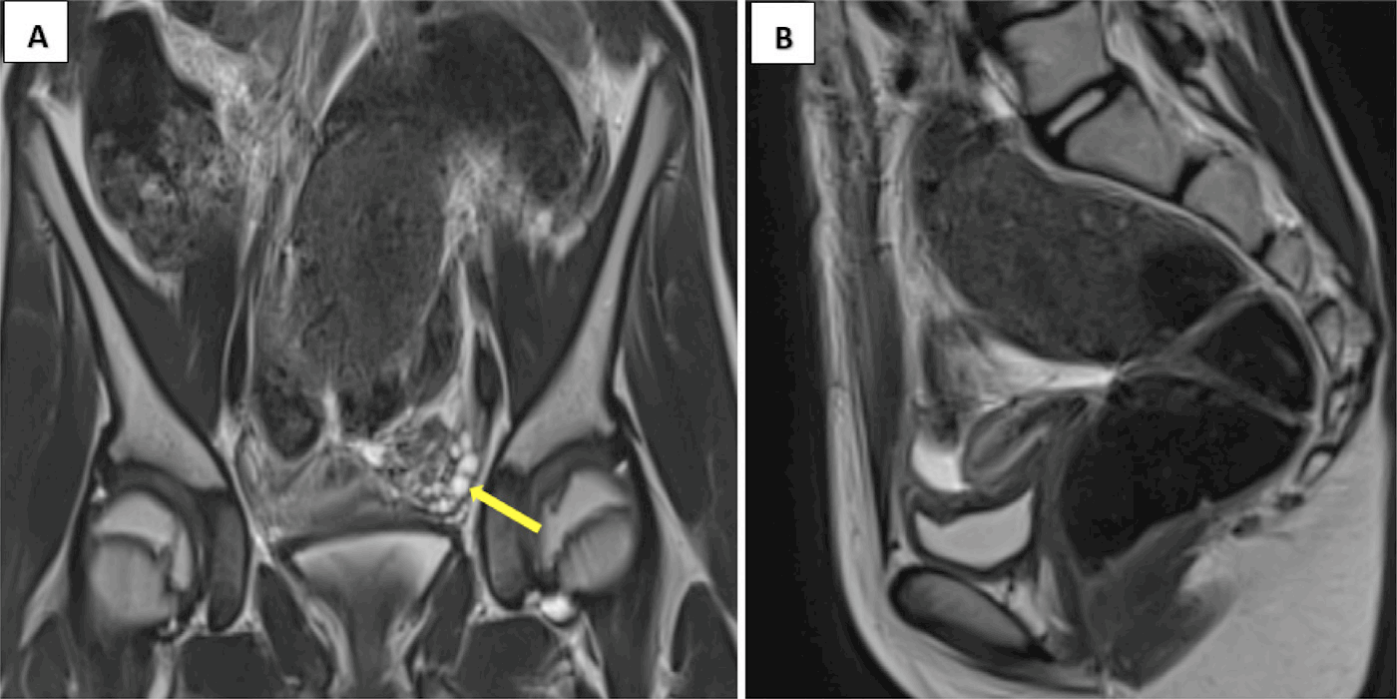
Postoperative abdominopelvic MRI. Coronal (A) and sagittal (B) views in T2-weighted sequences. The surgical bed appears clear, with no evidence of residual mass. The left ovary (yellow arrow) is of normal size and morphology, with a follicular pattern.
